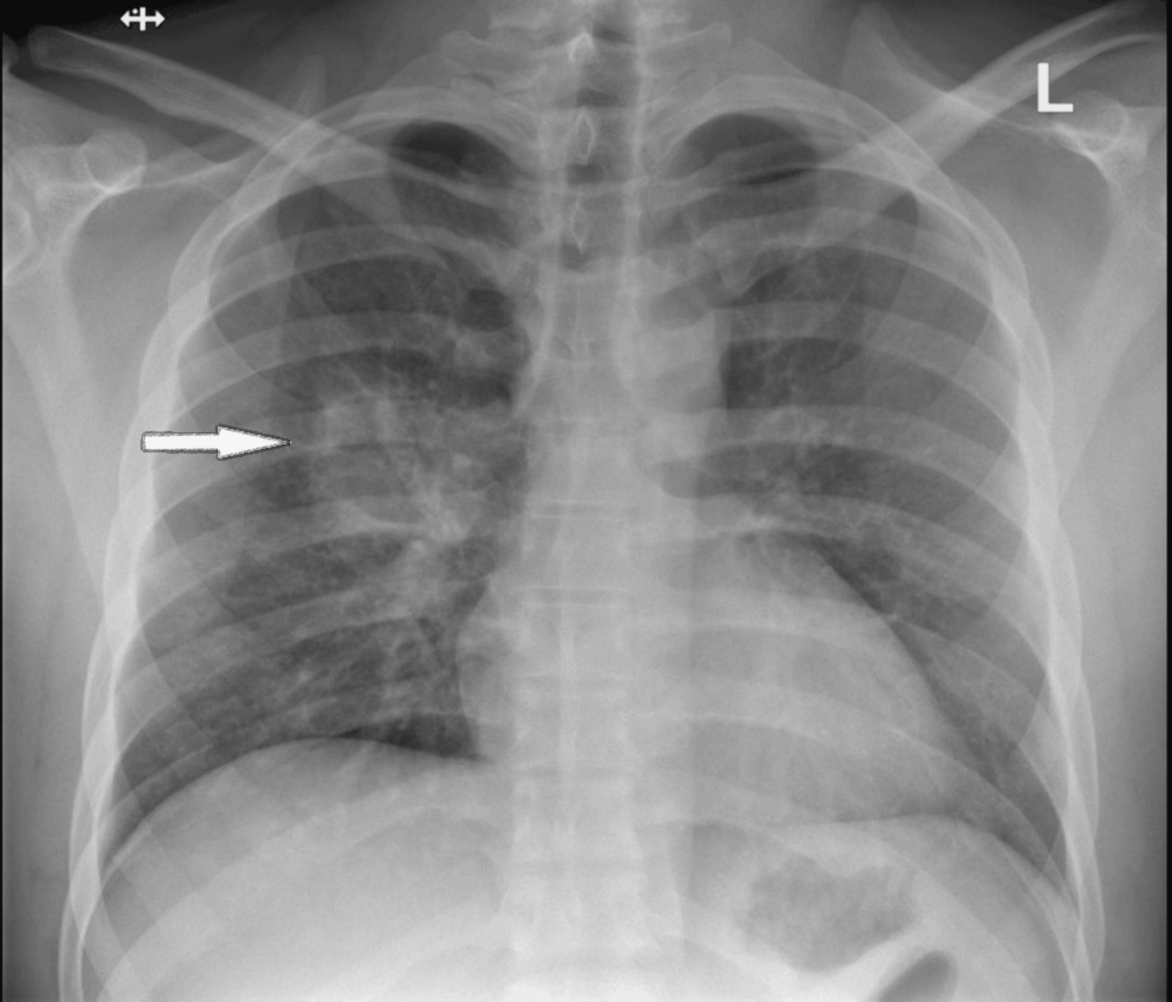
Chest X-ray showing a mass-like lung lesion located at the right hilum (arrow)
